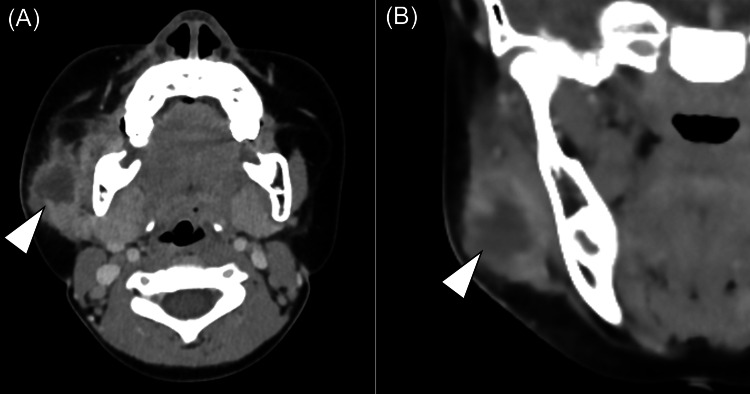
A contrast-enhanced CT scan (A) Axial image; B) Coronal image The abscess is seen in the buccal region (white arrowhead).
